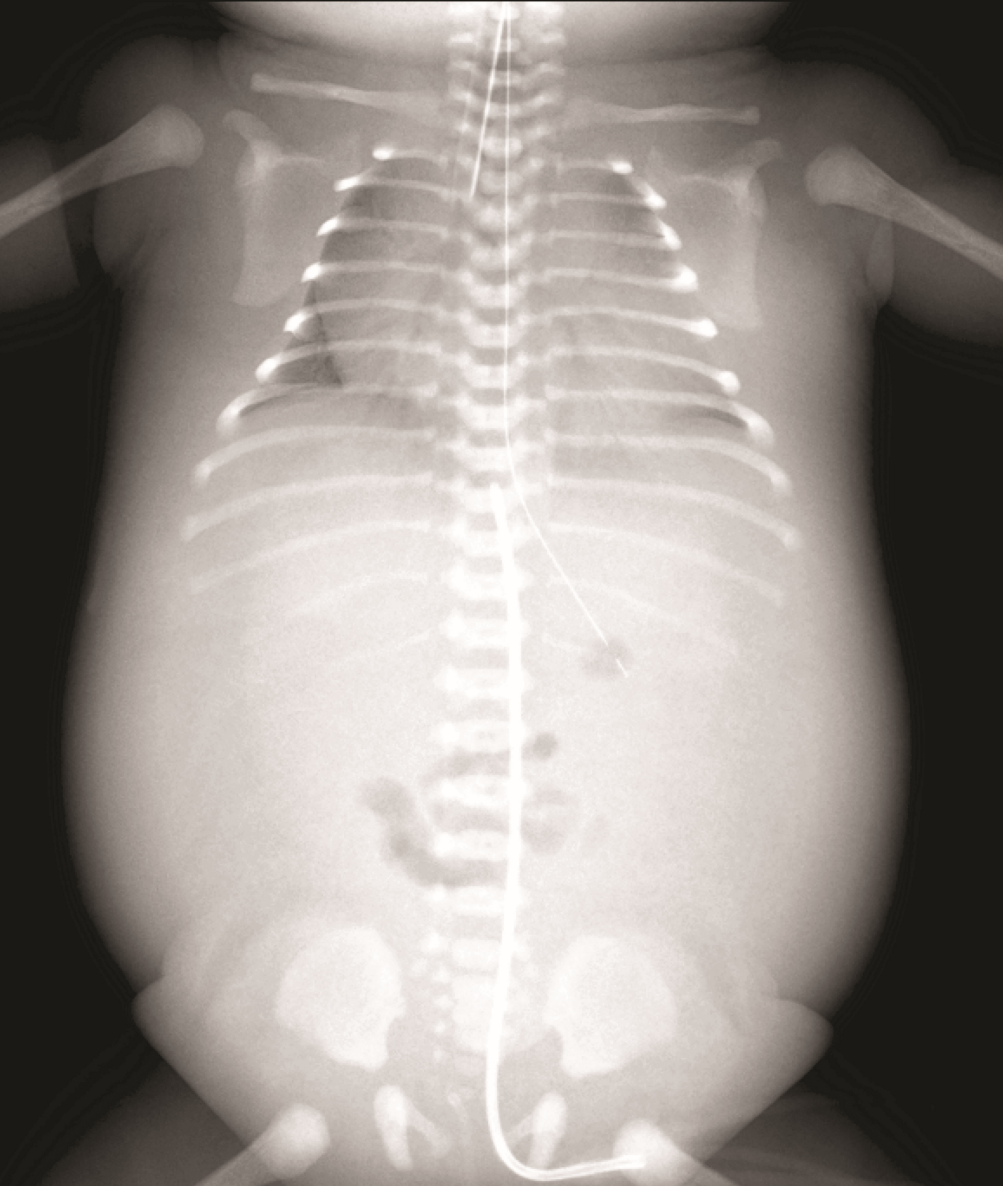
Chest and abdominal radiograph Chest and abdominal radiography performed on the first day of life revealed cardiomegaly, pulmonary hypoplasia, and marked systemic and pulmonary edema.
